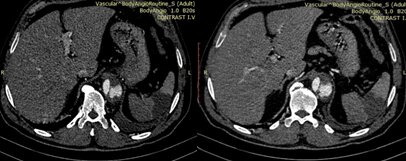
CT angiography 10 days after presentation: Type B aortic dissection and Celiac trunk dissection. One of the branches of the splenic artery is no longer patent, and there is an obvious splenic infarct.
